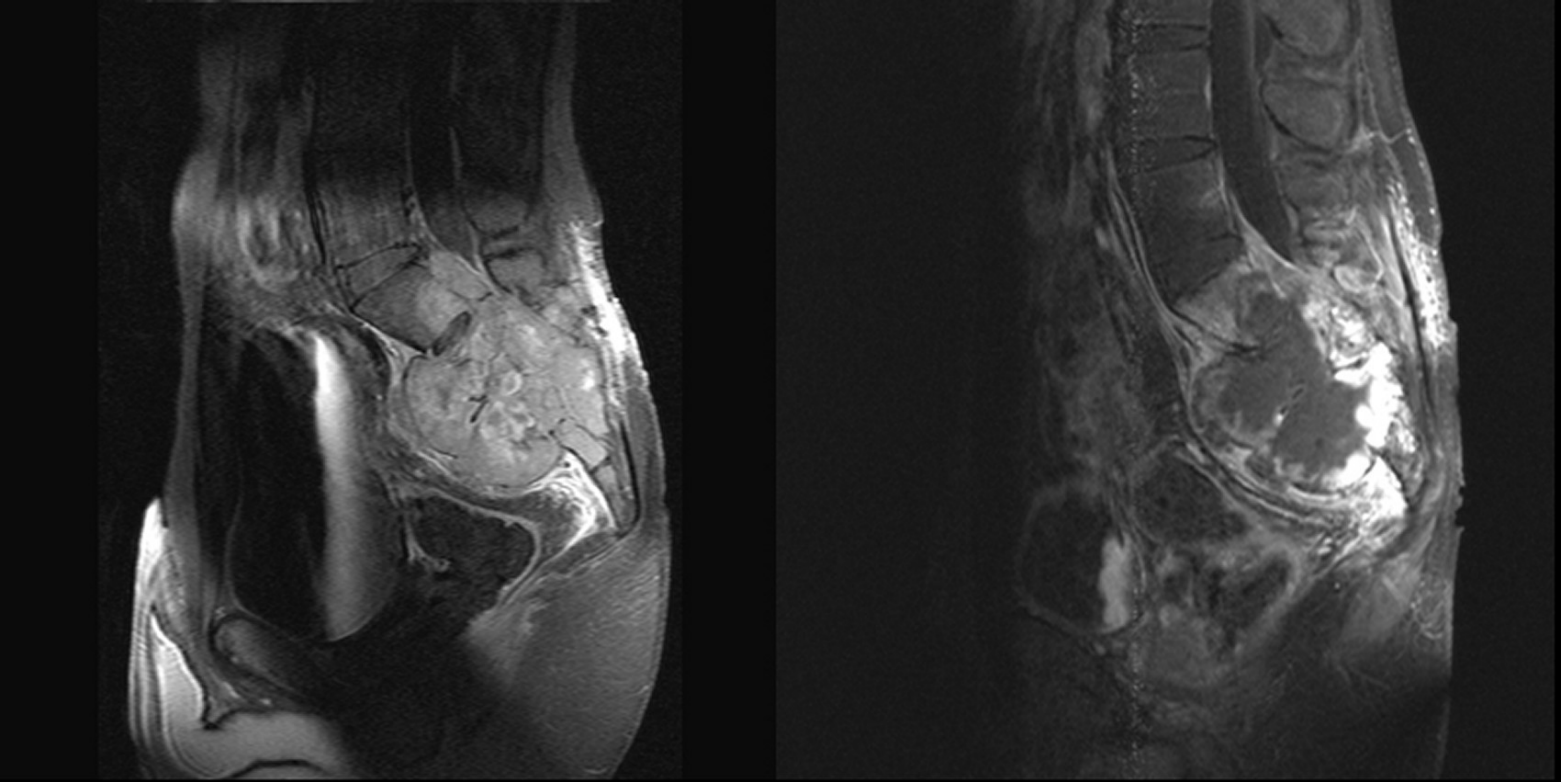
Development of central tumor necrosis in patient 4. left side: MRI before radiotherapy, right side: MRI 1 year after radiotherapy with development of massive central necrosis
